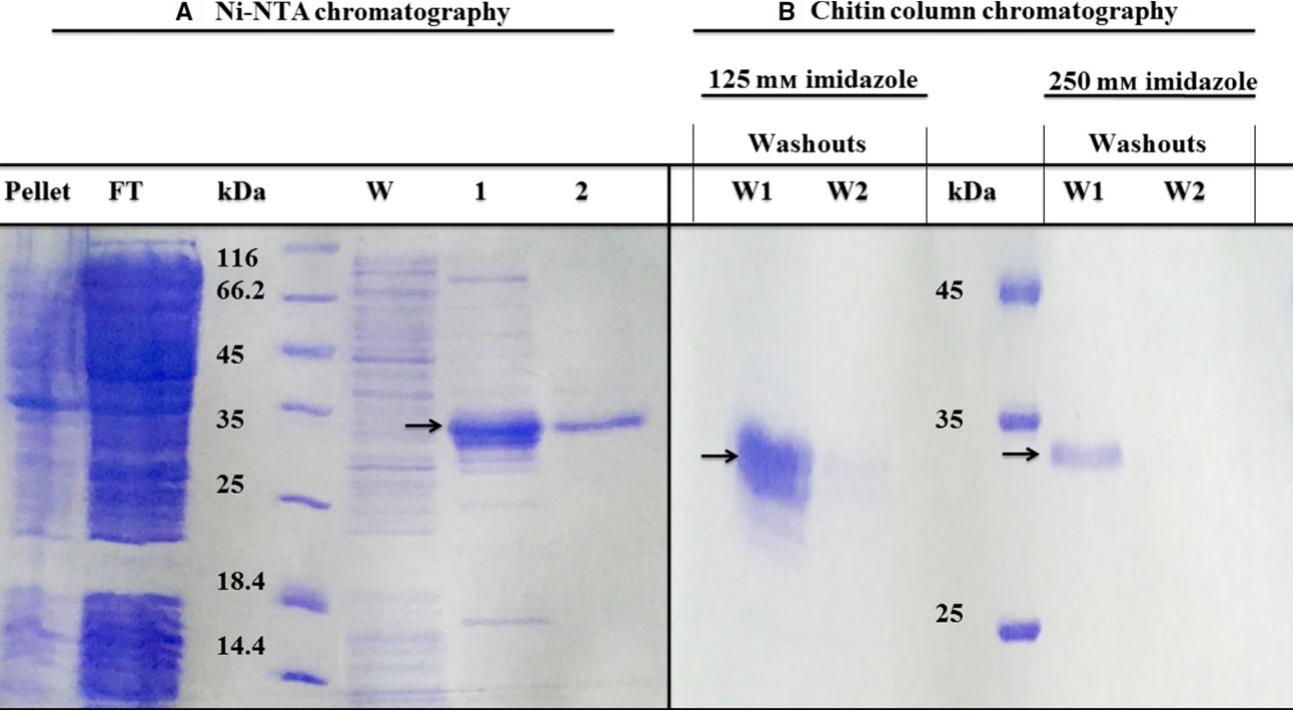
SDS/PAGE (12%) of the pellet obtained after ultrasonication and purified fractions of proteins obtained after (A) Ni‐NTA chromatography and (B) chitin column affinity chromatography. FT, flow‐through; W, washout; lane ‘1’: 125 mm imidazole eluate; lane ‘2’: 250 mm imidazole eluate. Arrows point to ^His^Dianthin‐EGF.
